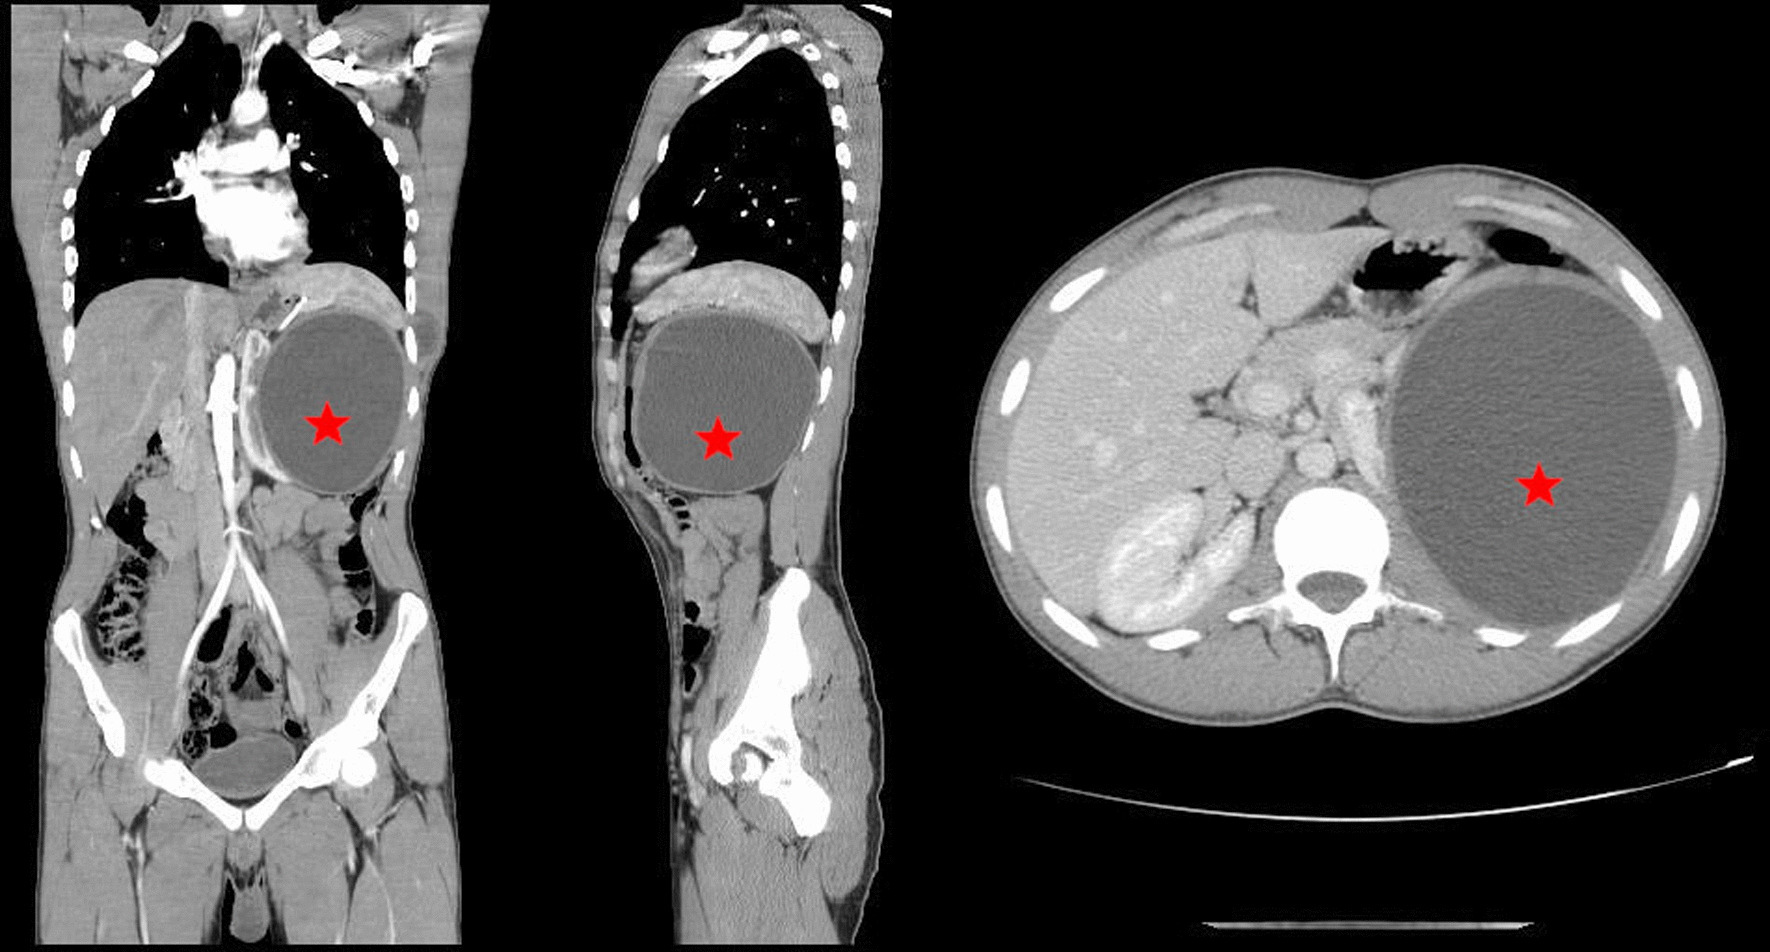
Contrast-enhanced CT (CECT) of the abdomen showing a large exophytic cyst (star) measuring 14 × 13.5 × 14 cm in the middle and upper pole of left kidney with no solid component or calcifications. Taken in all sections: coronal (left), sagittal (middle), and transverse (right)
